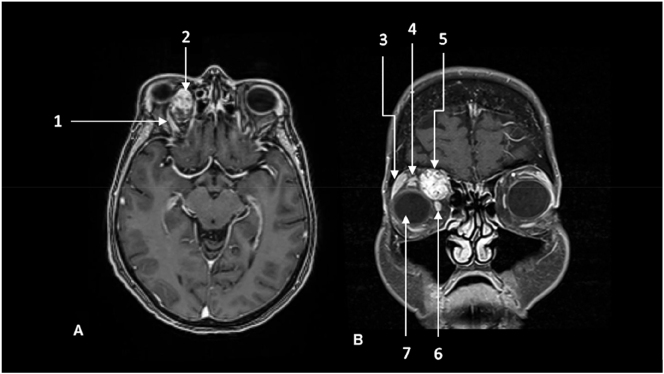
Preoperative MRI scans depicting the tumor of the right orbit. (A) axial T1-weighted sequence, 1 mm, 3D, contrast enhanced. Note the intraconal oval hyperintense tumor of the right nasal orbit with compression of the eyeball, optic nerve, orbital muscles and consecutive exophthalmos. (1) *M. rectus* superior, (2) tumor, (B) coronal T1-weighted sequence, 1 mm, 3D, contrast enhanced, with adequate delineation of the tumor. Further, note the edematous and compressed *M. rectus* medialis and *M. rectus* superior in contrast to the unaffected orbital muscles on the left. Further, note that *M. obliquus* superior is elevated and compressed superiorly and flattened against the periorbit and orbital roof. Note the dilated lacrimal gland on the right side in comparison to the left side as an indirect sign of a compression of the upper lid excretory ducts. (3) Lacrimal gland, (4) *M. rectus* superior, (5) tumor, (6) *M. rectus* medialis, (7) globe.
